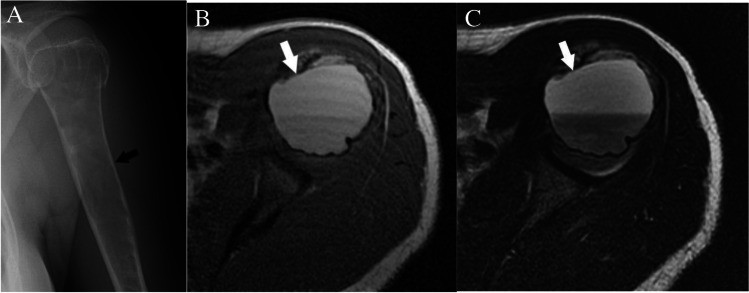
T1 hyperintense hemorrhagic lesions can mask underlying lesions and should follow the “High T2” algorithm. There is a lucent lesion (arrows) in the proximal humerus on the radiographs (A). The lesion has high signal (from hemorrhage) and a fluid level on the axial T1- (B) and axial T2- (C) weighted images. Lesion was was solitary bone cyst at definitive treatment
